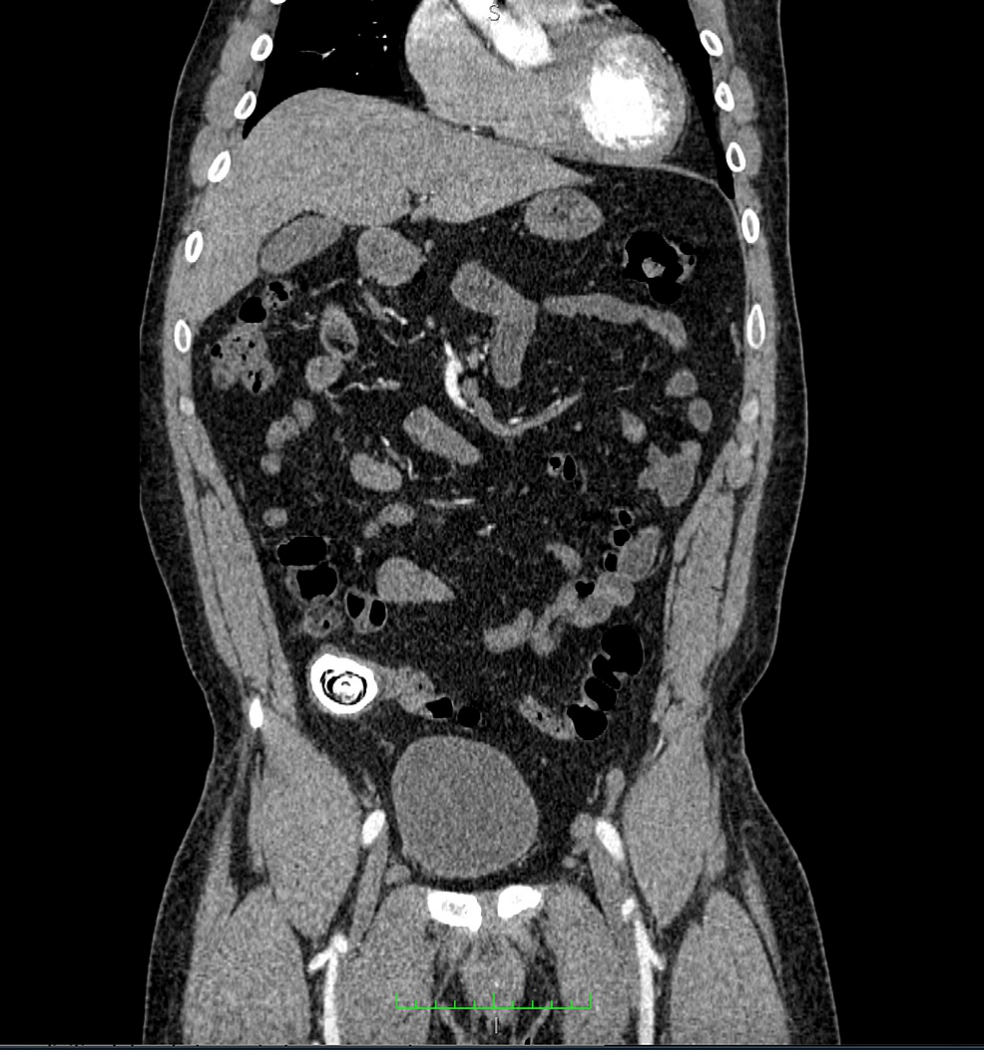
Coronal post IV contrast CT showing giant appendicolith within the right lower quadrant with periappendiceal fat stranding. No evidence of bowel obstruction or periappendiceal collection can be seen. CT: computed tomography
